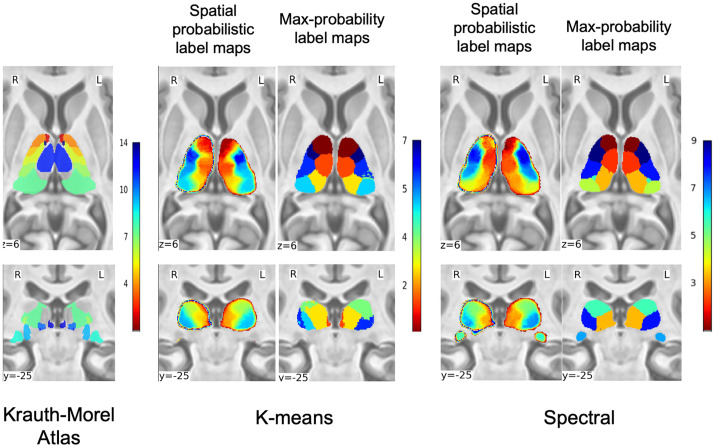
Qualitative Analysis of Thalamic Clustering Algorithms. Axial (z = 6 mm) and coronal (y = –25 mm) sections on the ICBM 2009b T1-weighted template comparing the Krauth-Morel atlas and thalamic parcellation strategy. For each method, the first column shows the spatial probabilistic label maps, and the second column shows the corresponding maximum-probability label maps.
